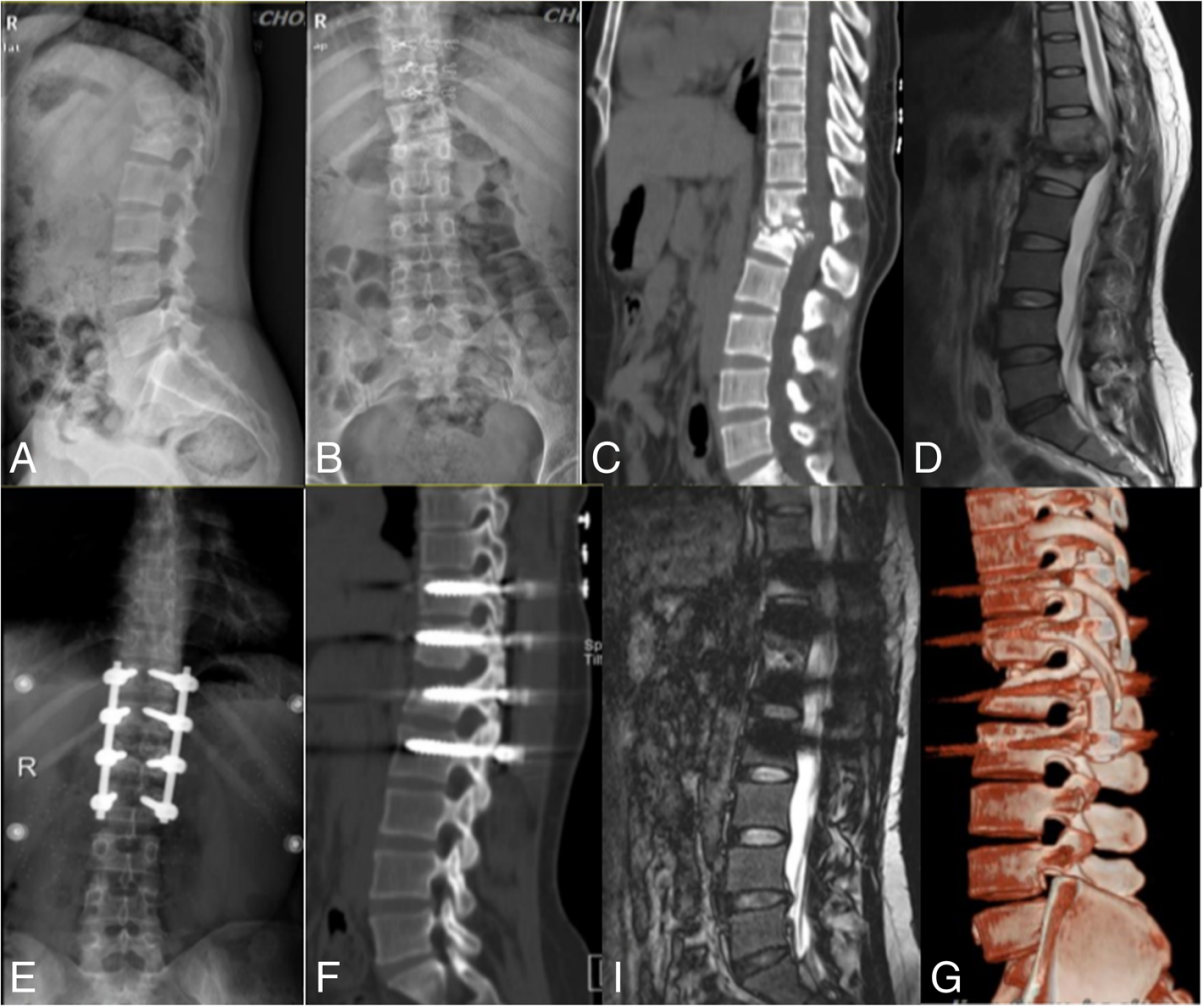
Single-stage posterior debridement, decompression and transpedicular screw fixation of a 35-year-old woman with thoracolumbar junction tuberculosis with associated neurological deficit. (a, b) Preoperative lateral and anteroposterior X-rays; (c) preoperative computed tomography (CT); (d) preoperative MRI; (e) X-ray at 3 days postoperative; (f) CT scan at 12 months postoperative; (i) MRI at 12 months postoperative; and (g) 3D reconstruction of CT at 12 months postoperative.
